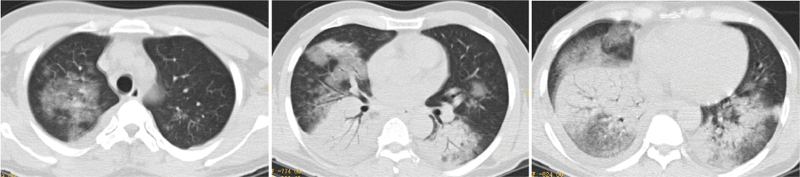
Chest computed tomography of Case 6, a 46-year-old male, showing large consolidation shadows and ground-glass opacity in both lungs, air bronchograms, and a small bilateral pleural effusion.
